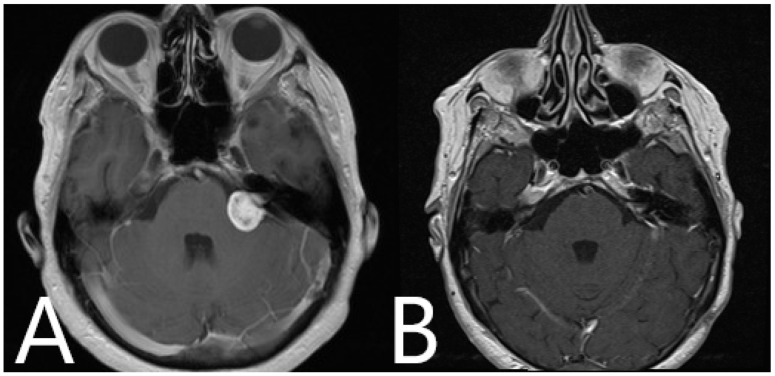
Patient No. 39. (A) Preoperative and (B) postoperative T1-weighted post-contrast MRI of the head shows subtotal resection of the tumor.
